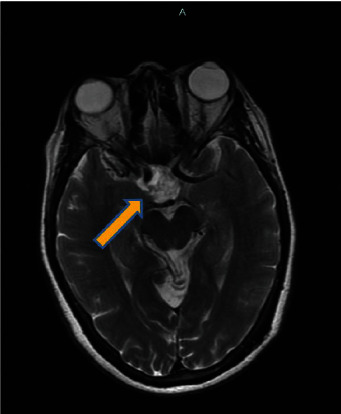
The axial T2-weighted image shows an enhanced mass measuring 1.9 cm × 2.1 cm with some cavernous sinus invasion.
